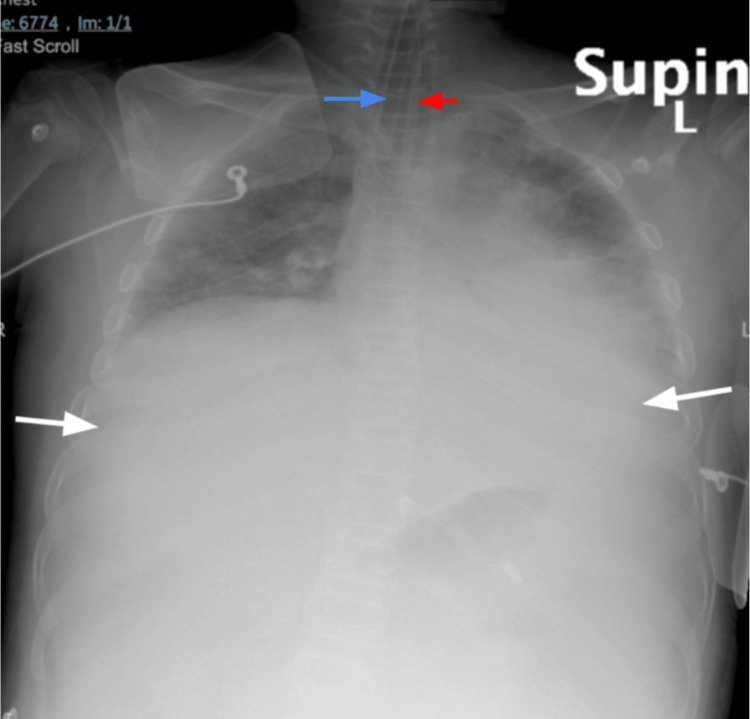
Supine chest X-ray showing pulmonary edema (white arrows), endotracheal tube (blue arrow), nasogastric tube (red)
